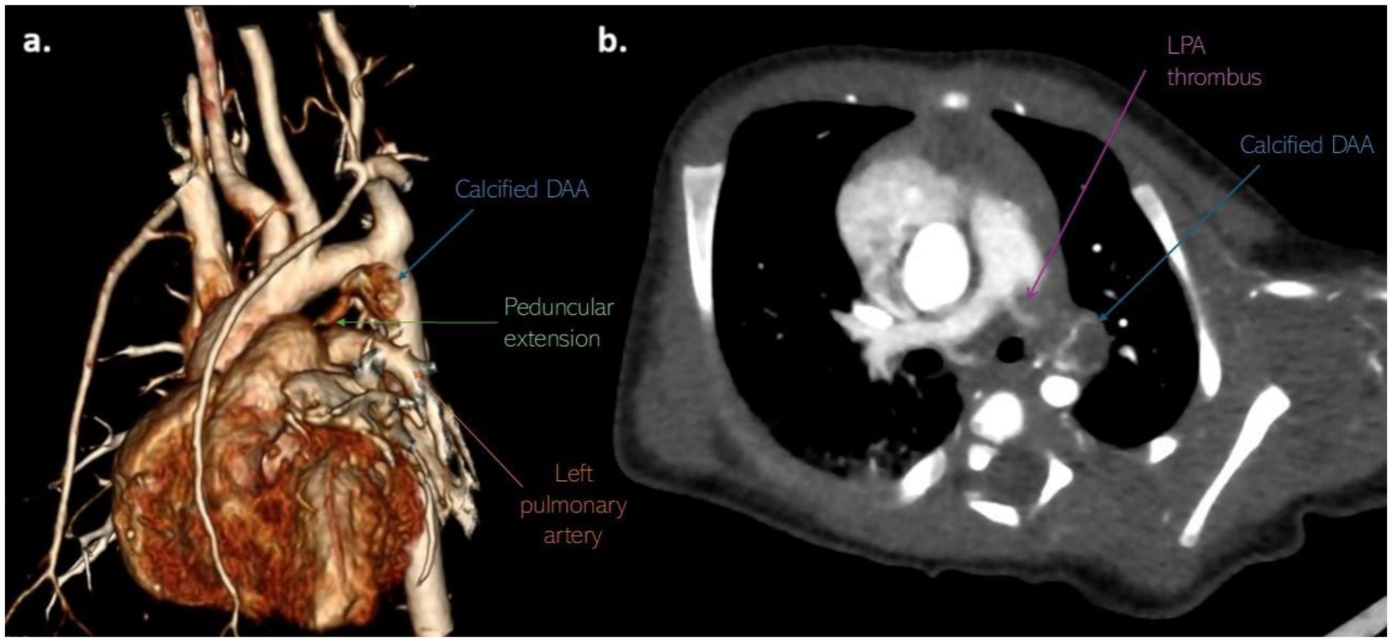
Non-gated CT angiography with (a) volume-rendered and (b) axial-oblique reconstructions show marginally calcified DAA (blue arrow) with peduncular extension (green arrow) toward the LPA, as well as thrombotic mass (purple arrow) in LPA. CT, computerized tomography; DAA, ductus arteriosus aneurysm; LPA, left pulmonary artery.
